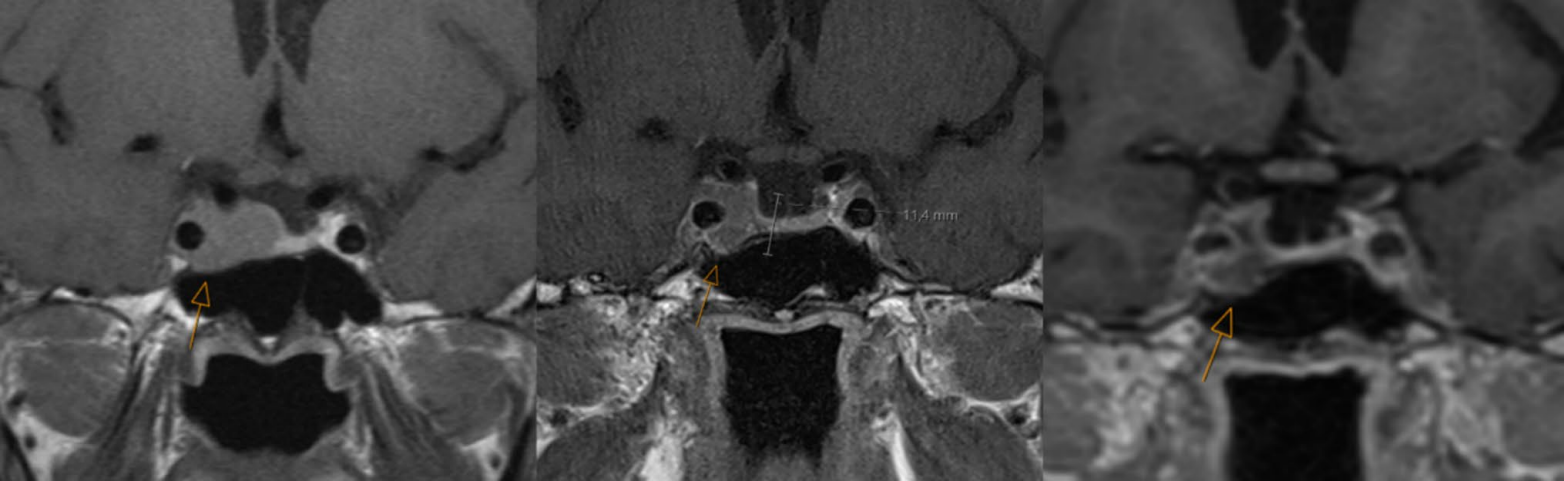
T1 weighted MR images enhanced with gadolinium. A patient who at baseline in 2010 (image to the left) had an invasive macroadenoma (arrow) and underwent primary surgery in 2012. He was not in remission and a residual adenoma was visualized (image in the middle, arrow). He had high IGF-1 concentrations and experienced side effects on medical therapy. Through a multi-disciplinary team meeting, he was referred to radiotherapy since surgery was inappropriate due to invasiveness. He was in remission after radiotherapy which he received in 2016. The image to the right from 2023 shows a finding (arrow) that is merely enhanced by gadolinium
